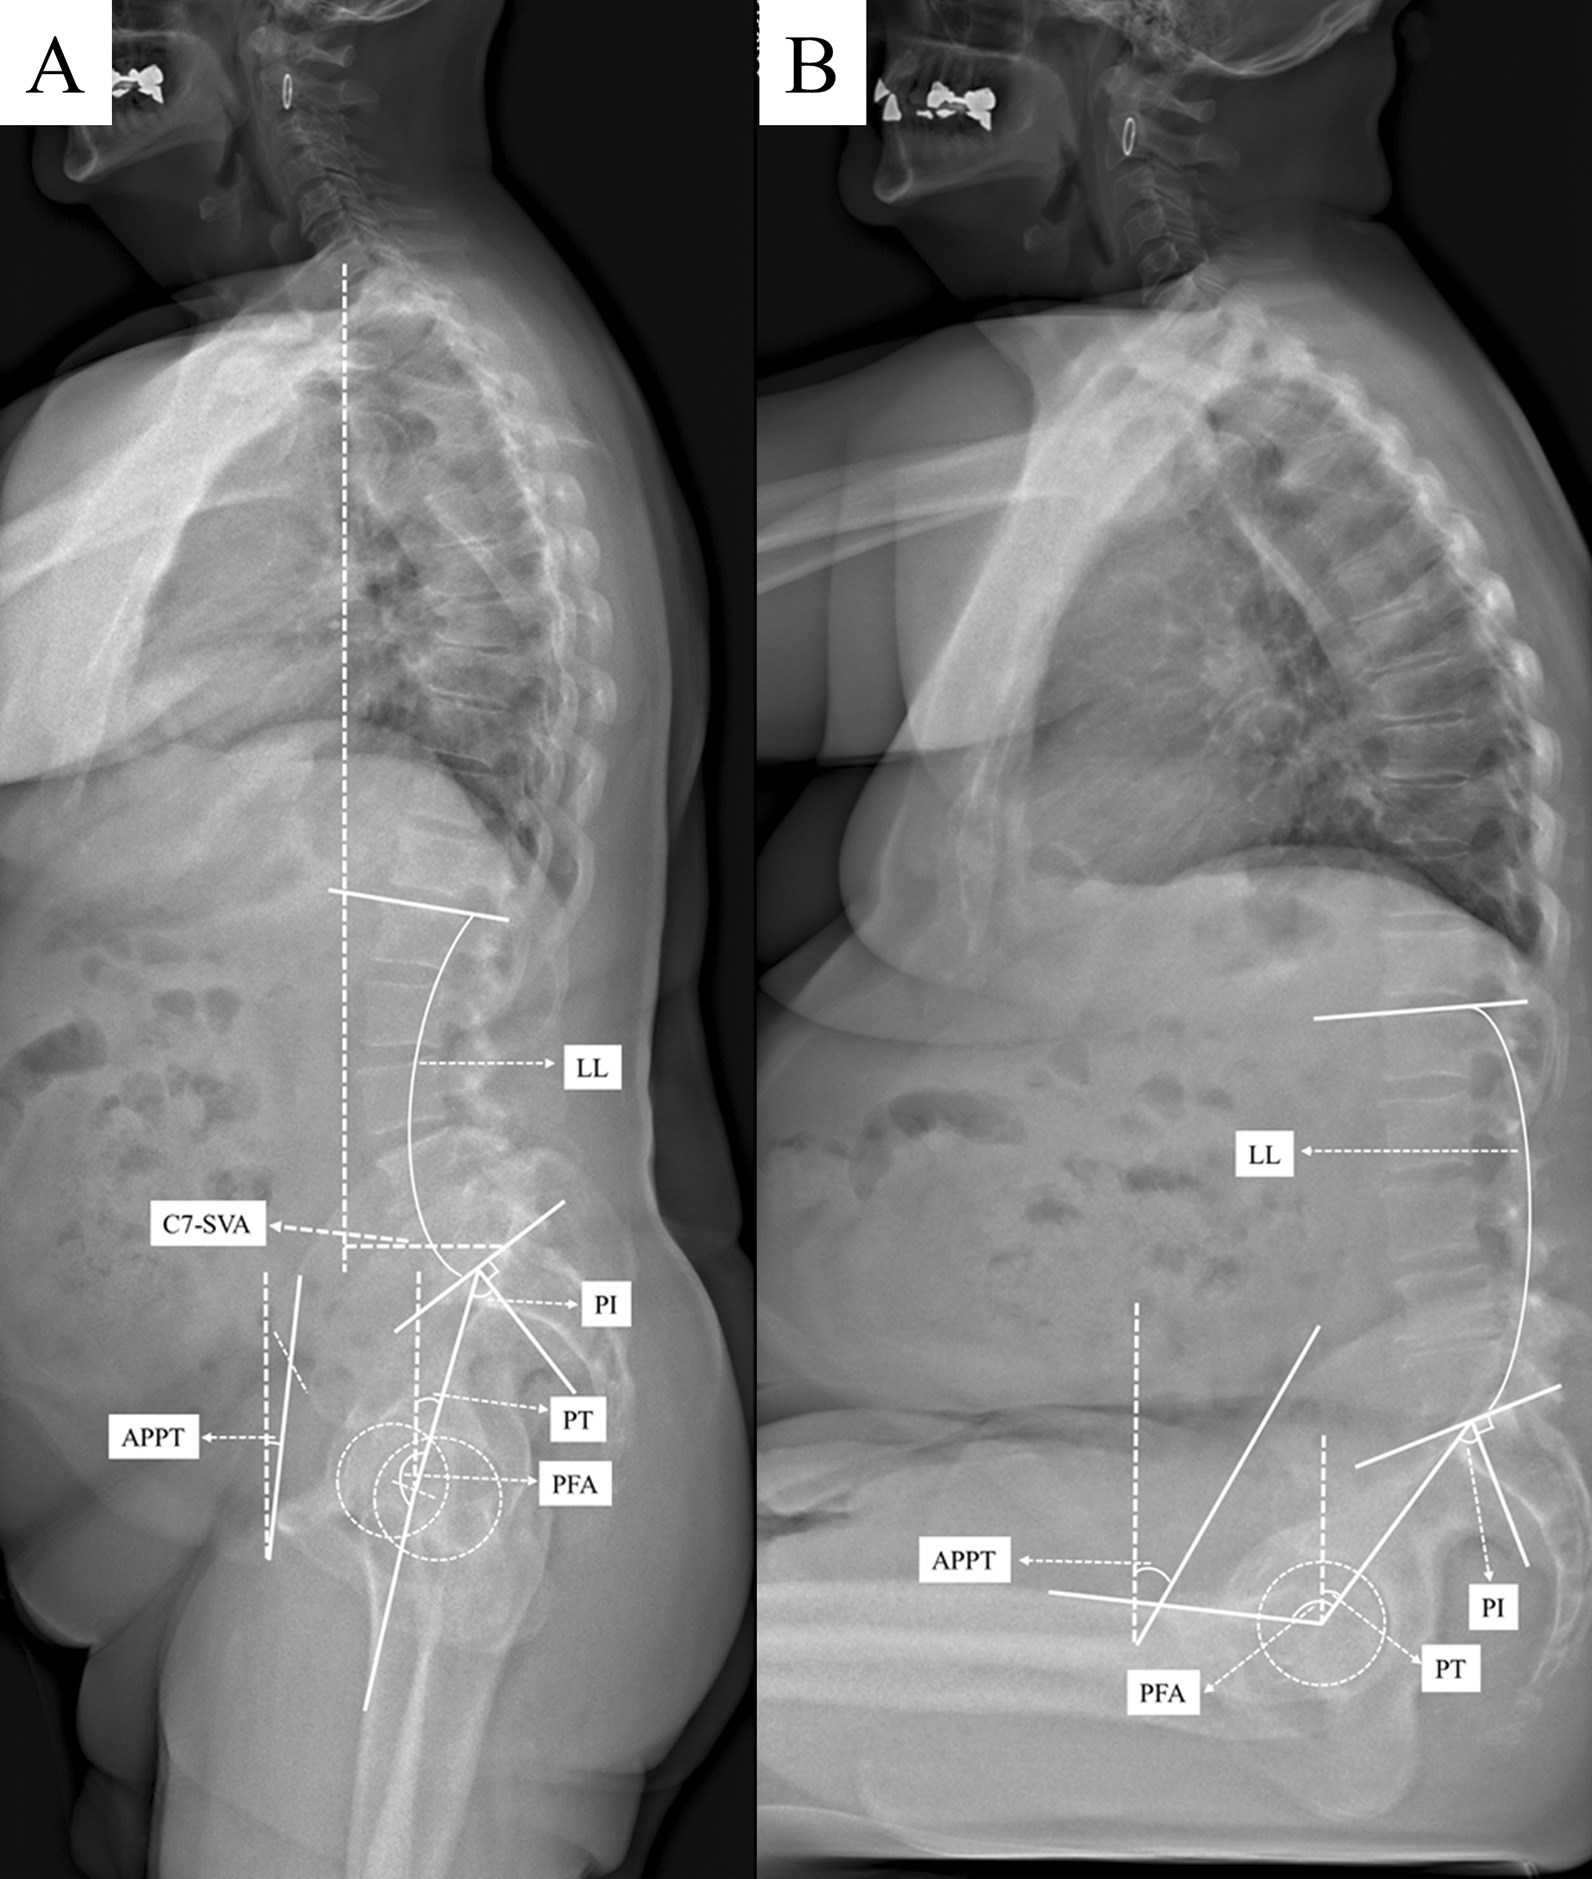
Sagittal standing (a) and sitting (b) EOS radiographs of the spine, pelvis and hip in a patient with obesity (BMI 36.3 kg/m^2^) depicting global spinal balance C7-sagittal vertical axis (C7-SVA) and spinopelvic parameter lumbar lordosis (LL),pelvic tilt (PT), pelvic incidence (PI), anterior plane pelvic tilt (APPT) and pelvic femoral angle (PFA)
